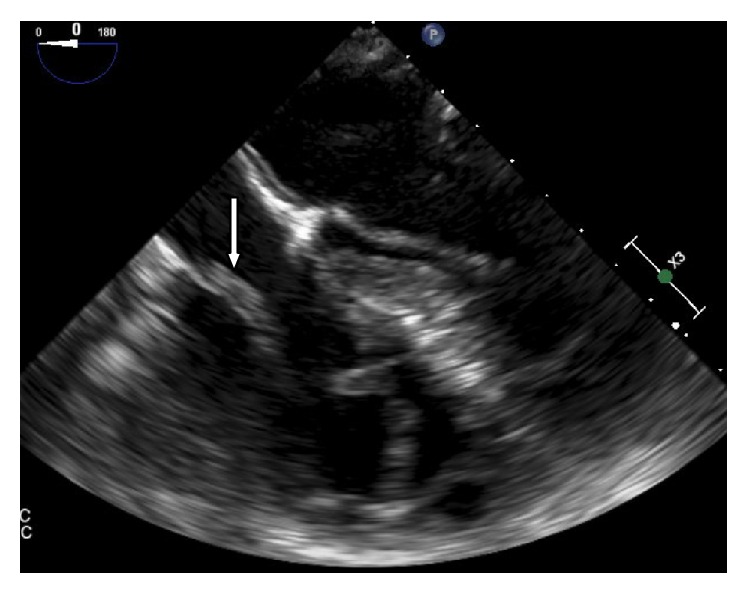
TEE image (apical four-chamber view) showing a serpiginous, elongated echodensity measuring 8.4 cm × 0.5 cm prolapsing into the tricuspid valve orifice deep into right ventricle; the attachment appears to be along the anterolateral right atrium.
